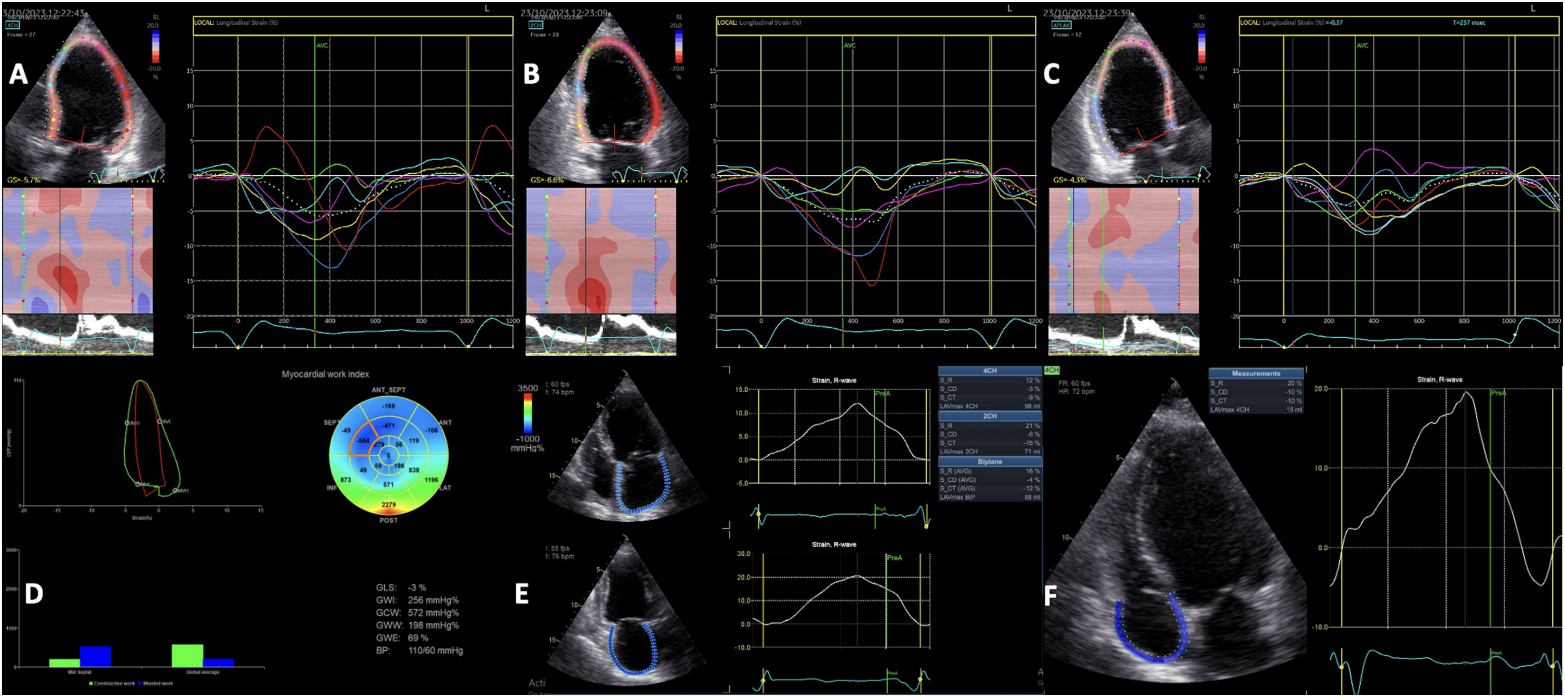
Two-dimensional speckle-tracking analysis of segmental left ventricular myocardial deformation showing the intraventricular dyssynchrony in the apical 4-chamber (A), 2-chamber (B) and 3-chamber (C) views. Myocardial work analysis showing increased GWW, and regional WW at the level of the septum, and low GWI, GCW and GWE (D) Left atrial strain analysis (E) and Right atrial strain analysis (F) showing reduced values in a patient with left ventricular dyssynchrony and left bundle branch block. GCW, global constructive work; GWE, global work efficiency; GWI, global work index.
